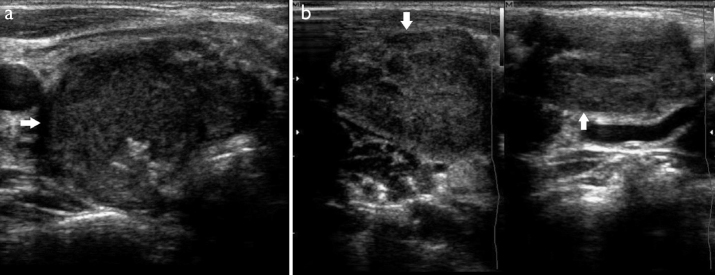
Neck ultrasonography. (a) Lymph nodes greater than 2 cm in the right lateral neck. (b) A 5.2 × 3 cm massive thyroid nodule with irregular borders covering a large part of the right lobe.
